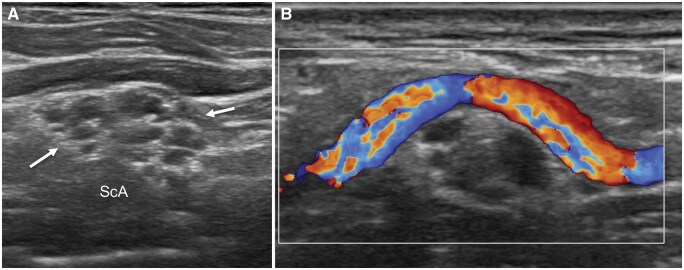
(A) Transverse ultrasound of the posterior triangle showing the “bunch of grapes” appearance of the divisions (arrows) aligned superomedial to the subclavian artery (ScA). (B) Transverse colour Doppler ultrasound shows the transverse cervical artery passing over the divisions of the brachial plexus.
